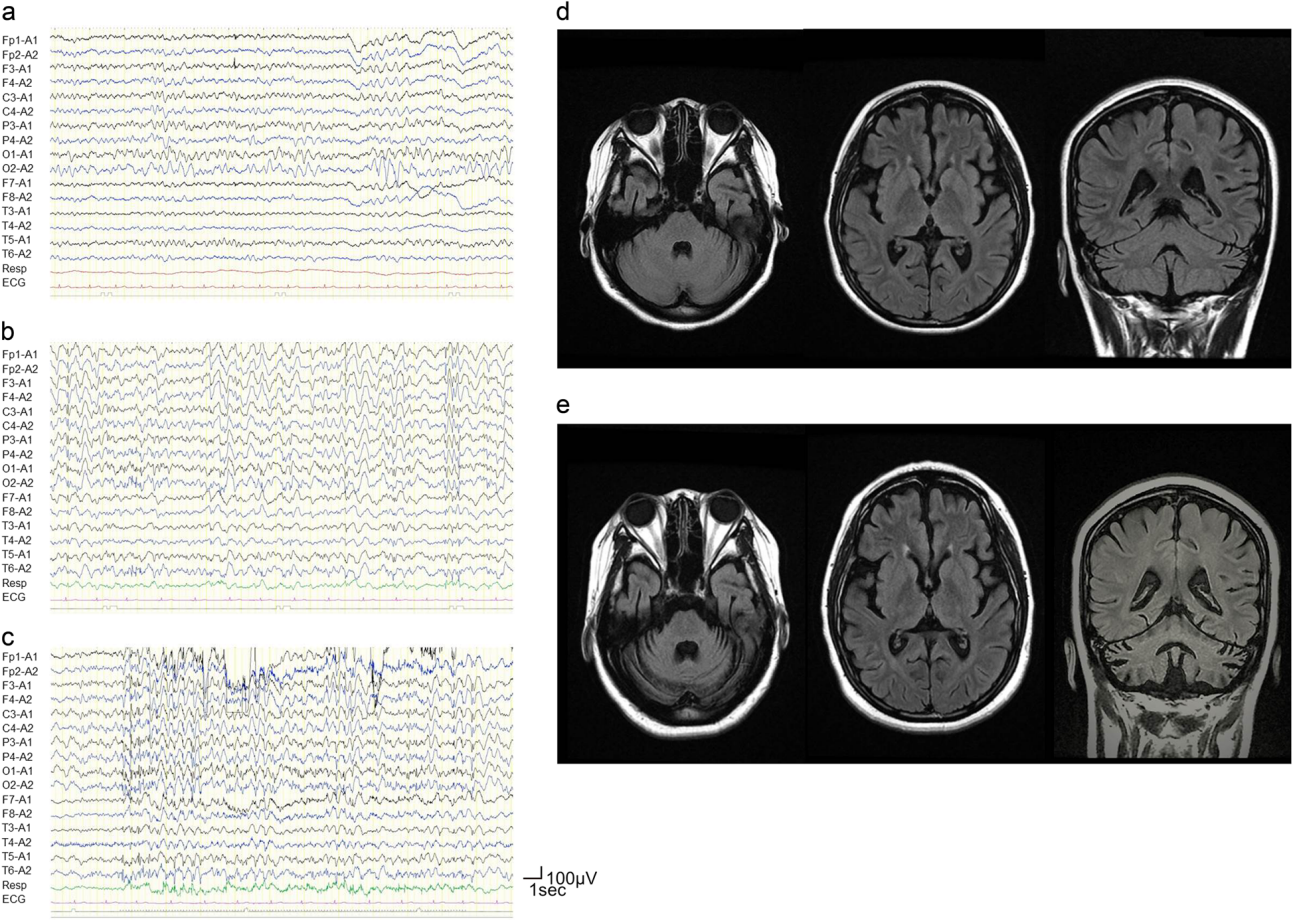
Electroencephalography and magnetic resonance imaging of the proband (III-4). a Continuous occipital slow waves were observed when she was 12 years old; b generalized spikes and slow waves, and c marked photosensitivity were observed when she was 14 years old. Brain magnetic resonance imaging (fluid attenuated inversion recovery) of the proband (III-4): d cerebral atrophy was prominent when she was 14 years old; e cerebellar atrophy appeared progressively when she was 17 years old
